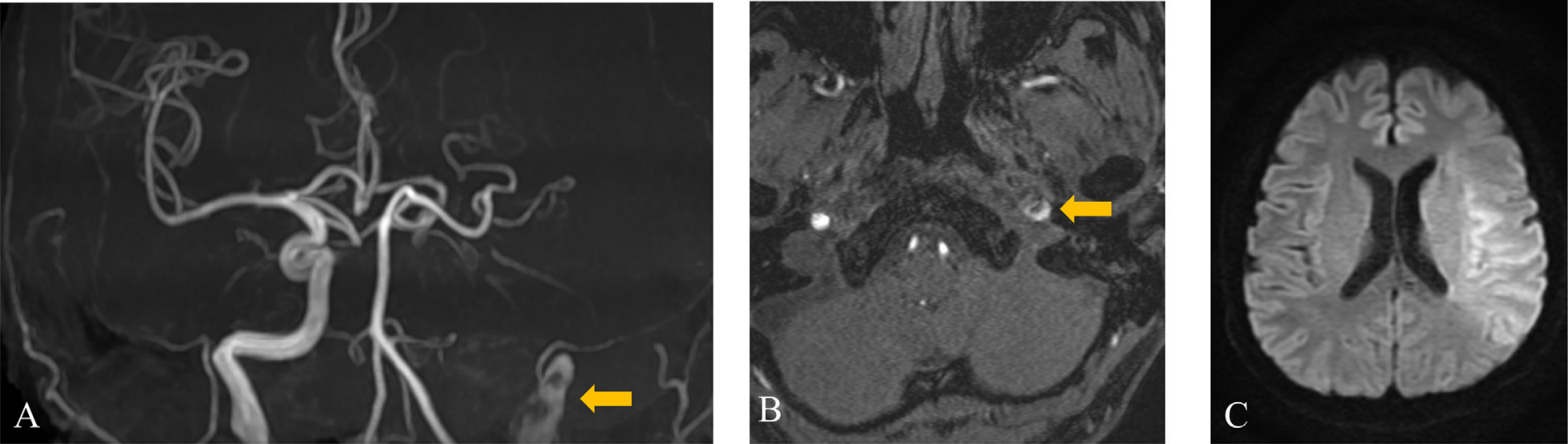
Preprocedure magnetic resonance imaging (MRI) of dissection of the internal carotid artery (ICA). (A and B) MRI revealed a subacute intramural hematoma in the wall of the right ICA (arrow). (C) MRI showed an acute ischemic lesion in the left cortex.
